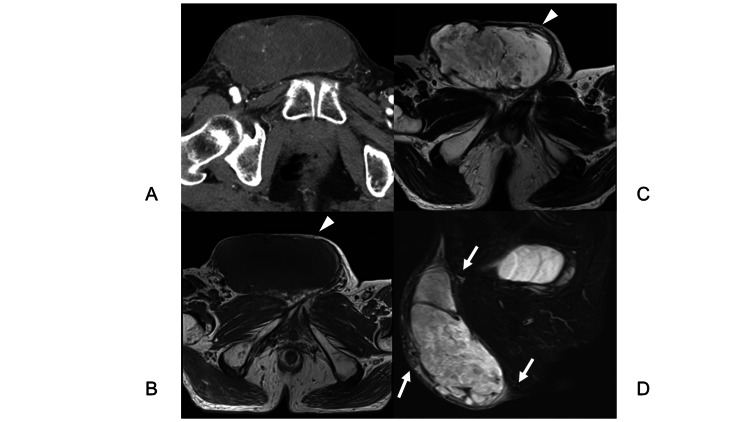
Contrast-enhanced CT and MRI of the pelvis (Case 3) Contrast-enhanced CT reveals an encapsulated subcutaneous 17.1×12.3×9-cm soft tissue tumor with numerous tumoral vessels (A: axial view). MRI reveals well-defined tumor (arrow heads) covered by a capsule is present within the subcutaneous fat tissue. The tumor exhibits iso-intensity on T1-weighted imaging (B: axial view), heterogenous high intensity on T2-weighted imaging (C: axial view) and enhancement on the distal side of the tumor on the gadolinium-enhanced T1-weighted fat-suppression image (D: sagittal view). Tortuous vascular structures (arrows) are proliferating around the tumor.
